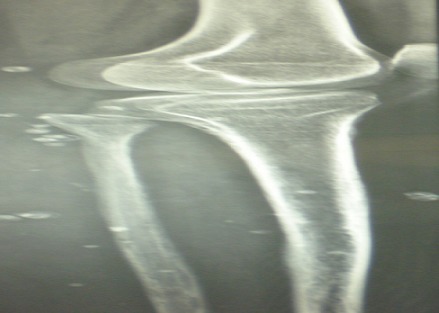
Radiographies standards de la jambe montrant des phlébolithes stigmates d'une malformation veineuses: Vue 2
